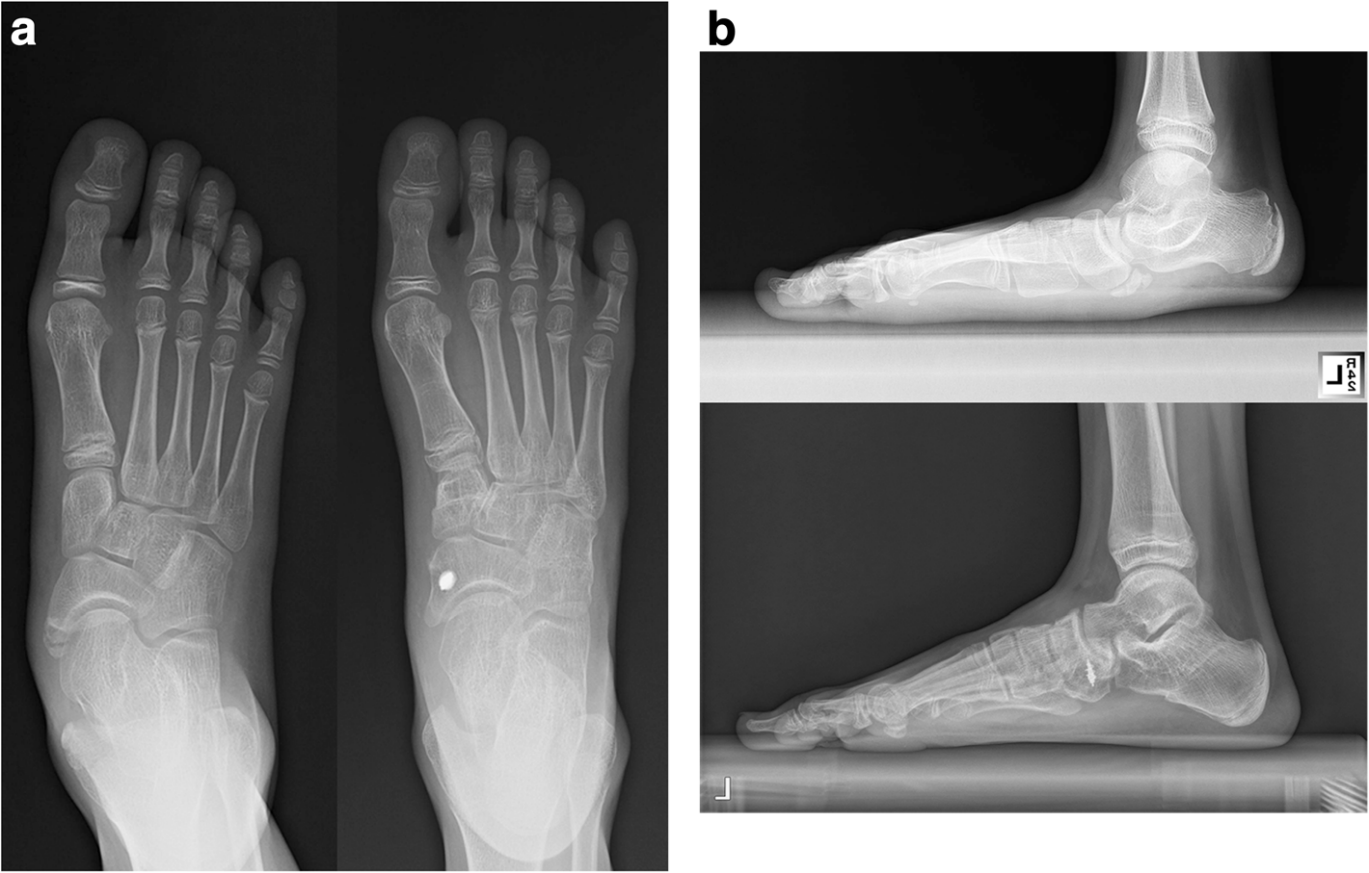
Comparative preoperative and 1-year postoperative radiographs of a 9-year-old girl. (a) Preoperative (left) and postoperative (right) weight-bearing AP radiographs demonstrating improvement of AP alignment. (b) Preoperative (upper) and postoperative (lower) weight-bearing lateral radiographs showing correction of plantar-flexed talus and restoration of longitudinal arch.
